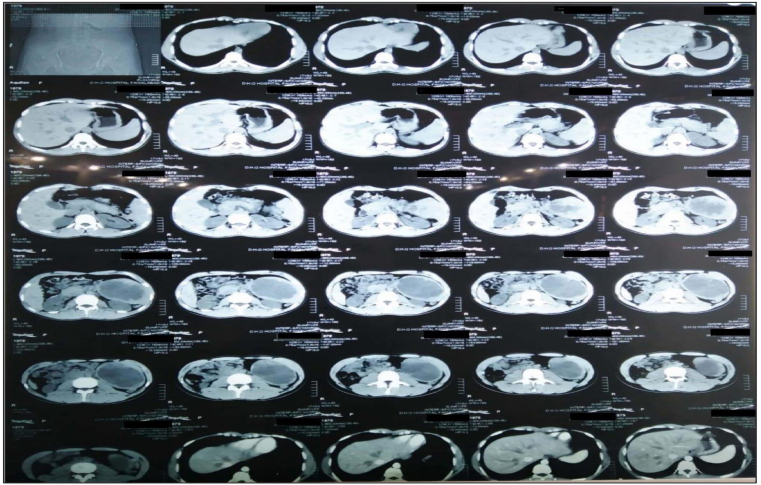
Computed Tomography scan of the abdomen with intravenous contrast enhancement showing the splenic patholog*y*.
